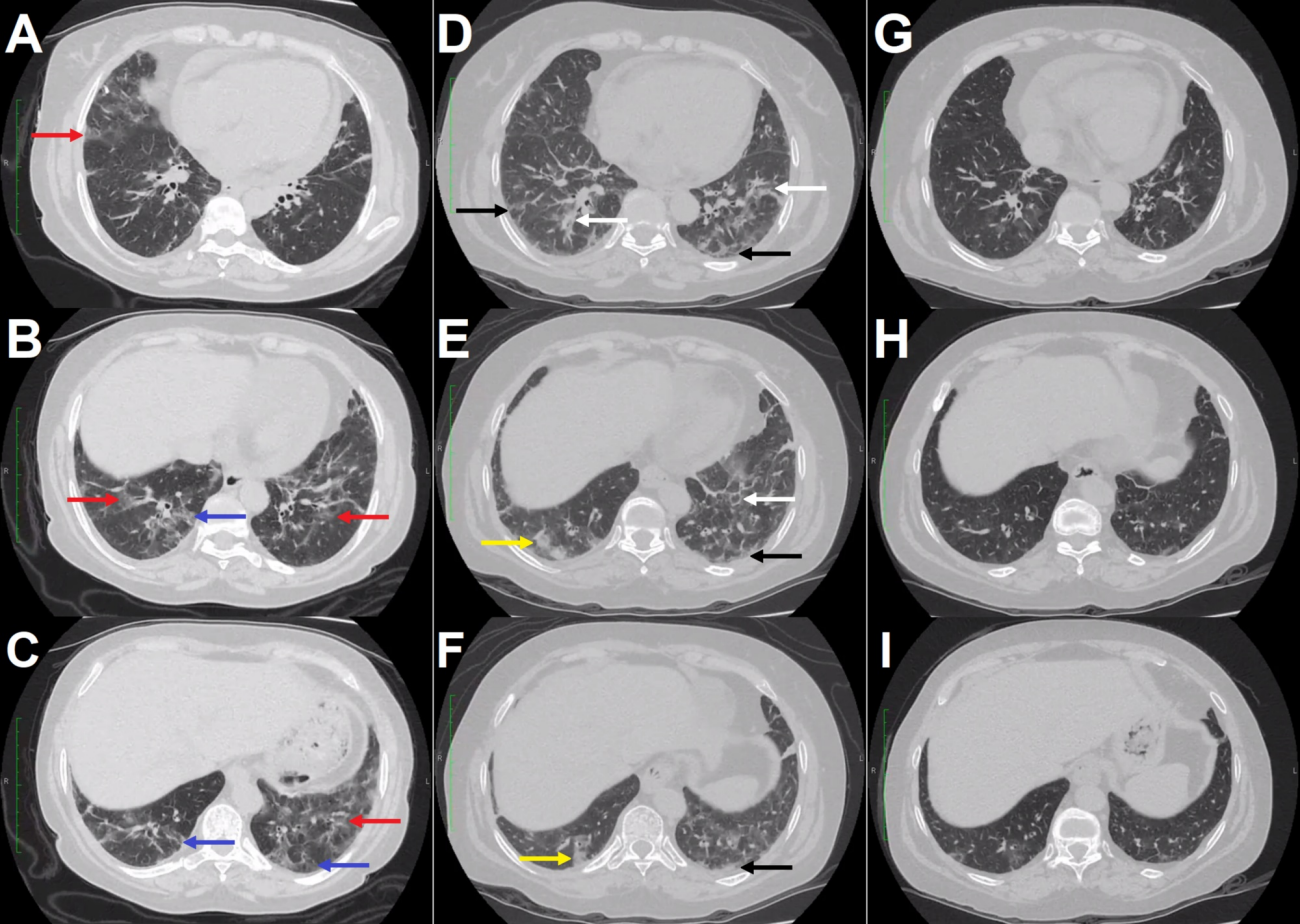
High-resolution computed tomography images of the patient with rheumatoid arthritis. Ground-glass opacities (red arrows) predominant in the lower third of the lungs, associated with mild traction bronciolectasis (blue arrows), suggesting nonspecific interstitial pneumonia (A-C). Increased areas of ground-glass opacities of a predominantly perilobular and peribronchovascular pattern (white arrows), associated with reticulation (black arrows) and bilateral patchy consolidations (yellow arrows), more evident in lower lobes, suggestive of organizing pneumonia (D-F). Significant reduction in ground-glass and consolidative opacities after tocilizumab suspension and cortiscorteroids onset (G-I).
